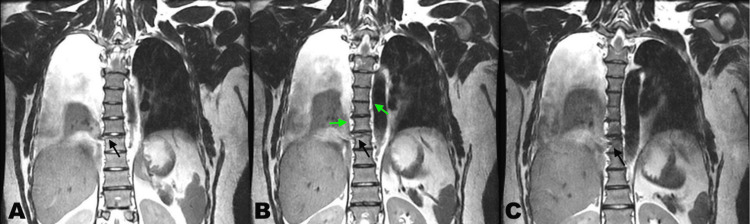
T2-weighted MRI demonstrating T8 chance fracture with associated thoracic duct rupture (black arrows) and ductal duplication (green arrows). Panels A-C represent three contiguous coronal T2-weighted slices arranged from anterior (A) to posterior (C). Black arrows indicate disruption of the thoracic duct, and green arrows demonstrate duplicated thoracic ducts present on both sides of the spine. A right-sided pleural effusion is present in all panels. The thoracic duct rupture and duplication are most clearly visualized in panel B. Images were obtained on a 3.0-T MRI scanner with fat suppression.
